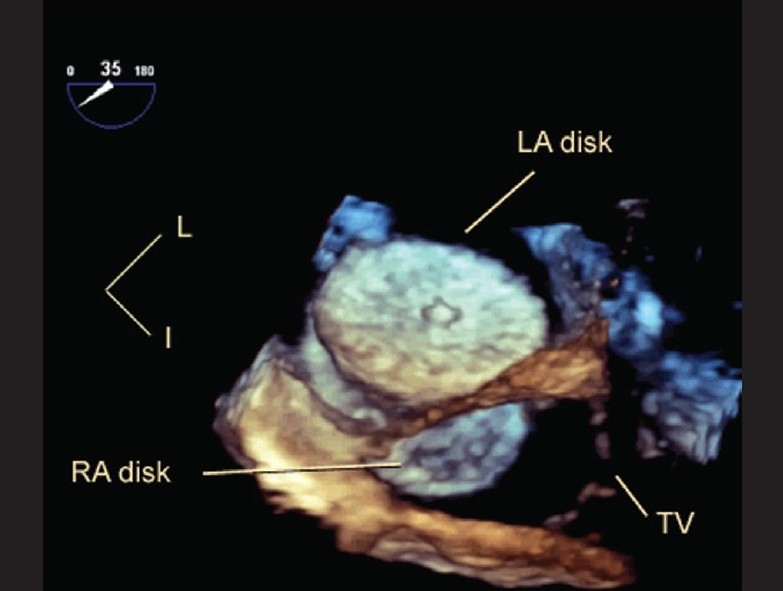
This is a live 3D transesophageal echocardiogram immediately following device closure of a fenestrated atrial septum with a cribriform Amplatzer septal occluder. The viewer is ‘virtually’ placed inside the left atrium, looking rightwards and posteriorly. The entire left atrial disk of the device is seen, with its central umbilication and the meshwork of metal that constitutes its frame. Part of the inferior portion of the right atrial disk (RA disk) and the tricuspid valve apparatus (TV) are also seen. I, inferior; L, left
